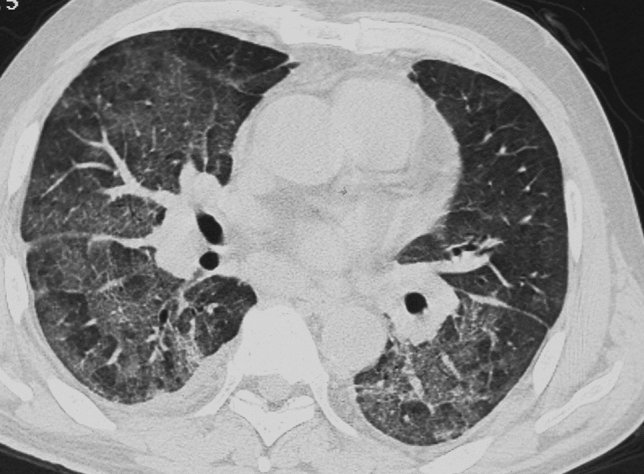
A 60-year-old man with pneumocystis pneumonia under treatment for acute lymphoid leukemia. High-resolution computed tomography shows extensive ground-glass attenuation with a mosaic pattern
